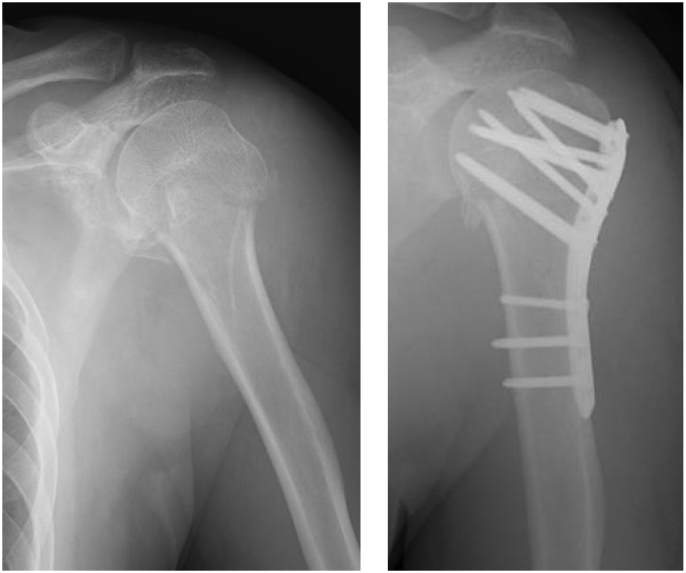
Radiographs: Proximal humeral fracture, pre and post-surgery.
